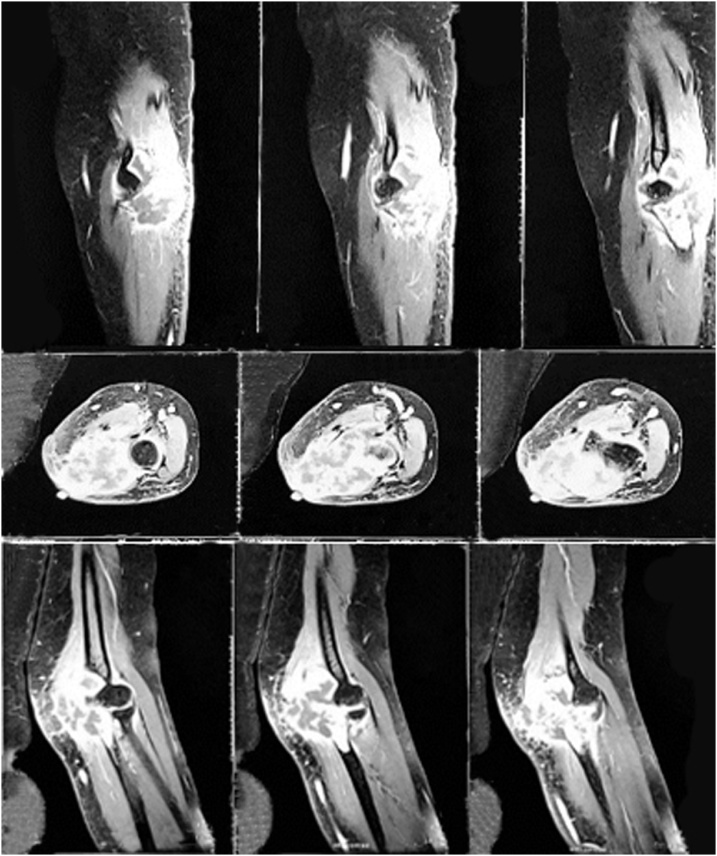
Pre operative MRI of right forearm. Hyperintense heterogen mass was seen at proximal ulnar bone that involves pronato teres, flexor digitorum superficialis, and fleksor carpi ulnaris muscles. The mass caused irregularity, necrotic area, and destruction of the proximal ulnar bone which suggested a malignant lesion.
